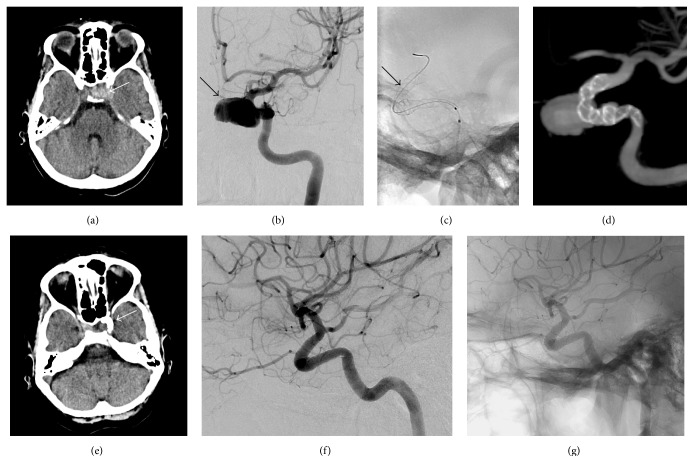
Cranial nerve palsy (patient number 1). 55-year-old woman presented with left oculomotor palsy. Cranial CT (a) showed hyperdense mass in the ophthalmic segment of the left carotid artery. Wide-necked intracranial aneurysm was proved by digital subtraction angiography (DSA, b) and the patient was treated using a flow diverter (c, d). In follow-up, clinical symptoms improved; the mass (e) and DSA confirmed no filling of the aneurysm sack (f, g).
